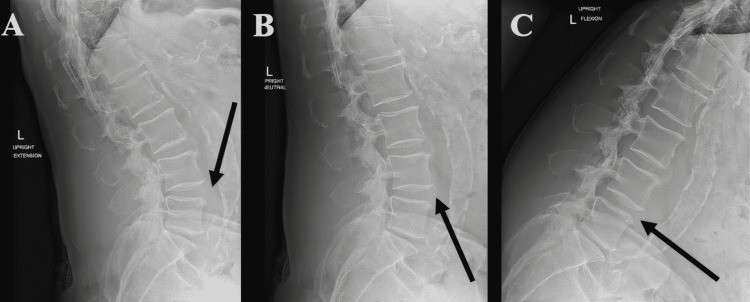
Preoperative X-ray of the lumbar spine Extension view (A) neutral view (B) and flexion view (C) of the lumbar spine with L4/5 grade I spondylolisthesis and diffuse spondylosis arrow: L4-L5 grade 1 spondylolisthesis
